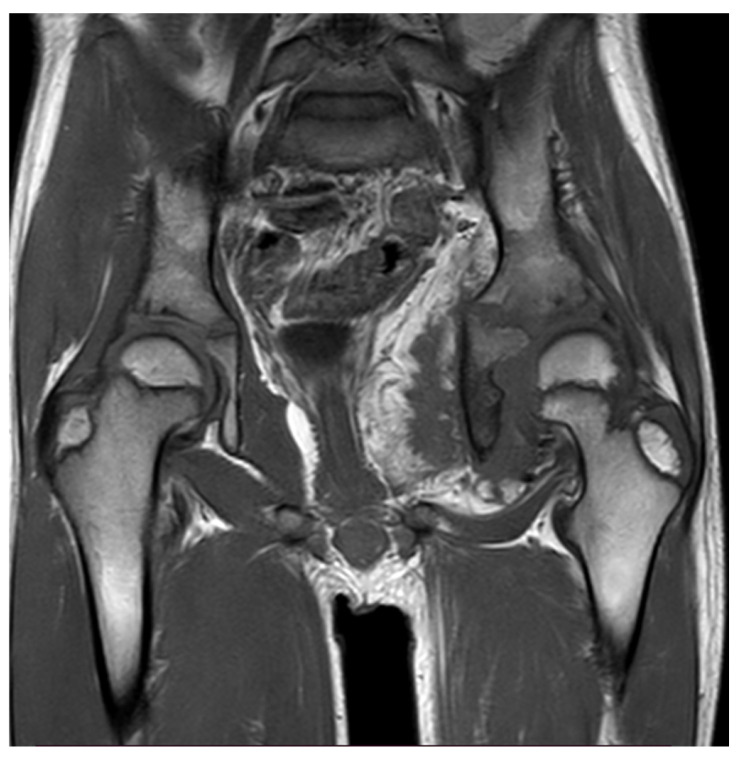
The MRI showing large heterogeneous soft-tissue mass in the abdominal cavity, with extension to the hip joint.
